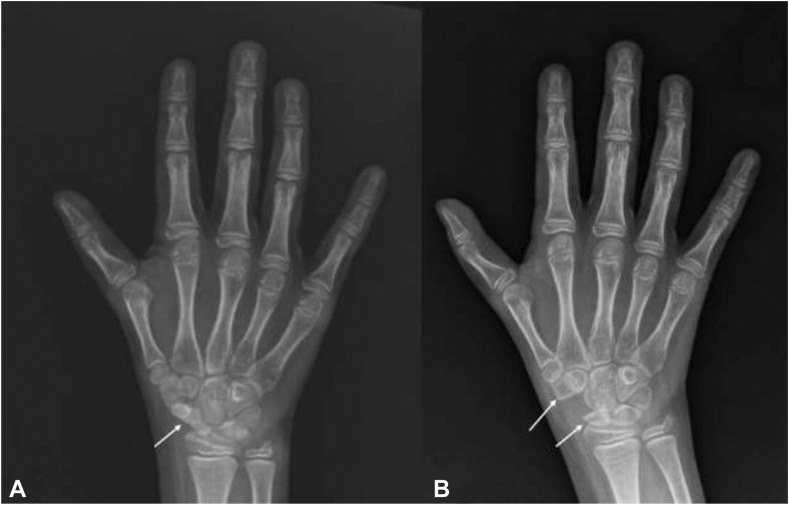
A Baseline preoperative radiograph of the right hand with an arrow demonstrating the region of flattening and sclerotic appearance of the scaphoid with interface irregularity at the trapezium, indicative of nonosseous coalition. B Six-month postoperative radiograph of the right-hand wrist depicting scaphotrapezial coalition resection along with partial carpectomy of the proximal trapezium and distal scaphoid (arrows).
